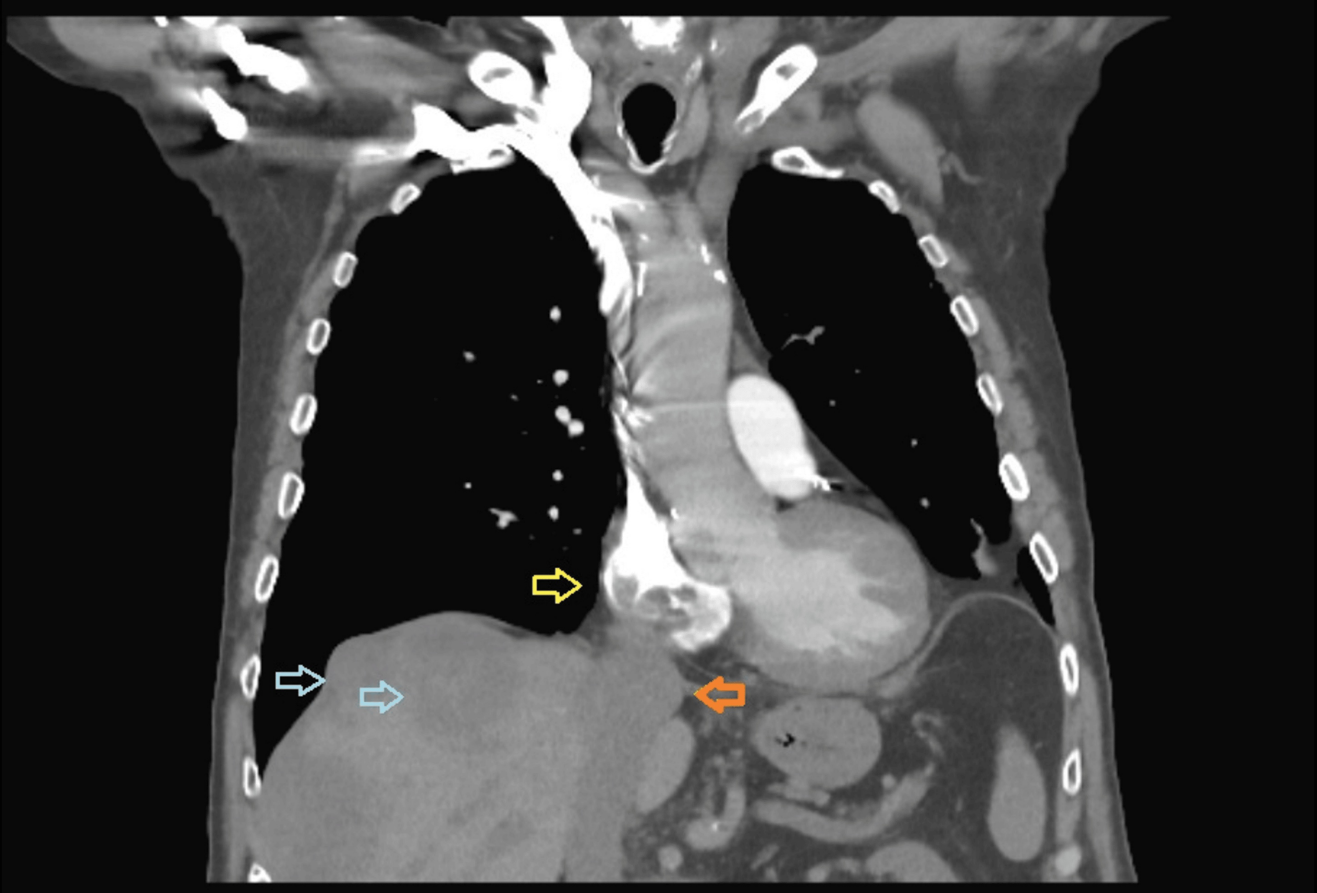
CT chest with contrast, coronal section CT scan showing the right atrial extension of the tumor thrombus. The yellow arrow indicates filling defects in the right atrium due to the tumor thrombus. The blue arrows indicate hypoattenuating hepatic lesions. The orange arrow indicates the distended inferior vena cava (IVC) with the tumor thrombus.
